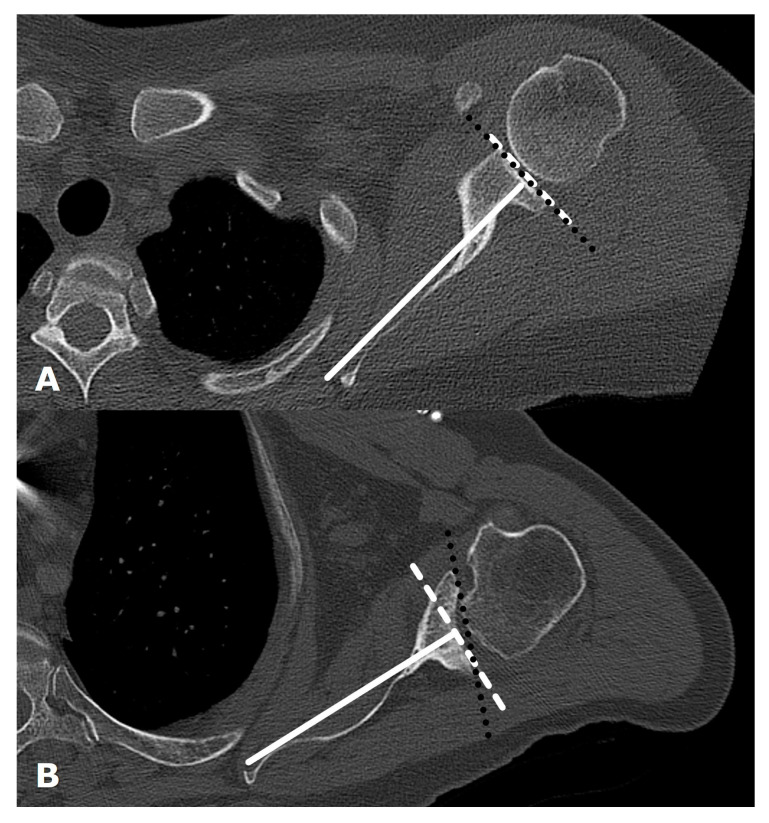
Axial CT images from two different patients (A). Normal glenoid version. (B). Retroversion secondary to glenoid erosion from osteoarthritis. A line (solid white) is drawn from the superior medial scapular border to the center of the glenoid and a line (white dashed) is drawn perpendicular to this on the axial image at or just inferior to the tip of the coracoid. Glenoid version is the angle formed between the perpendicular line (white dashed) and a line (black dotted) drawn along the glenoid articular surface.
